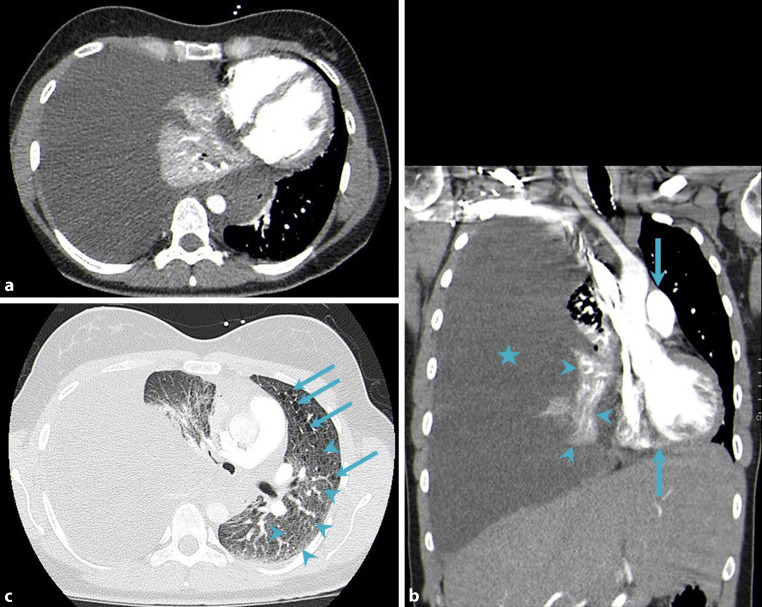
CT of the chest. Axial (a) and coronal (b) images (soft tissue window setting); massive right pleural effusion (*asterisk*), atelectasis of the right middle and lower lung lobes (*arrowheads*) as well as a marked mediastinal shift to the left (*arrows*). Axial image (c; lung window setting); multiple small lung cysts (diameter up to 6 mm; *arrows*) as well as extensive ground glass opacities (*arrowheads*)
